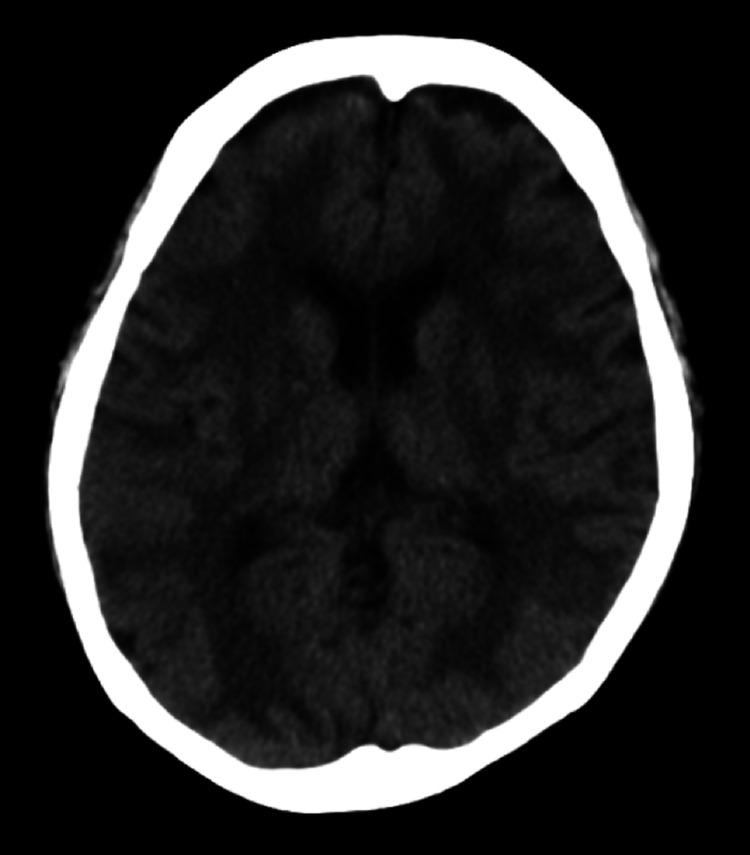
Head computed tomography shows diffuse hypodense subcortical regions.
